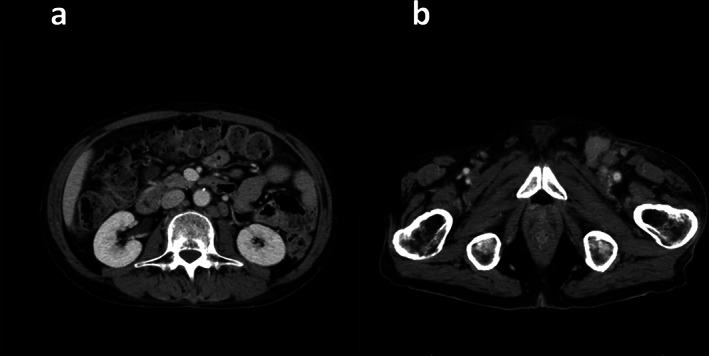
Computed tomography of the abdomen shows enlargement of multiple intraperitoneal lymph nodes, such as the para‐aortic lymph nodes (a) and inguinal lymph nodes (b)
